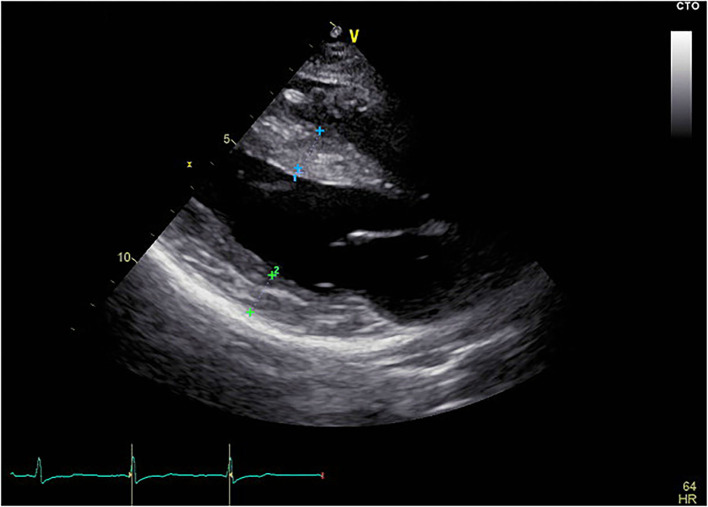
Echocardiogram of the patient. The echocardiogram showed thickened interventricular septum and left ventricular posterior wall. Blue cross marked part: interventricular septum, thickness was 1.4 cm; Green cross marked part: posterior wall of left ventricle, thickness was 1.4 cm; Yellow scale bar shows length in cm.
